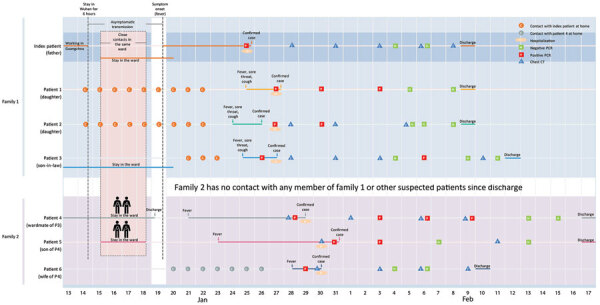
Chronology of a 2-family cluster of severe acute respiratory syndrome coronavirus 2 infection, including travel and contact history, in familial and hospital settings, Xuzhou, China, January 13–February 17, 2020. Dates of case confirmation, hospitalization, and discharge are labeled. Real-time fluorescent reverse transcription PCR for severe acute respiratory syndrome coronavirus 2 infection and corresponding results are indicated, together with the dates of chest CT. CT, computed tomography.
